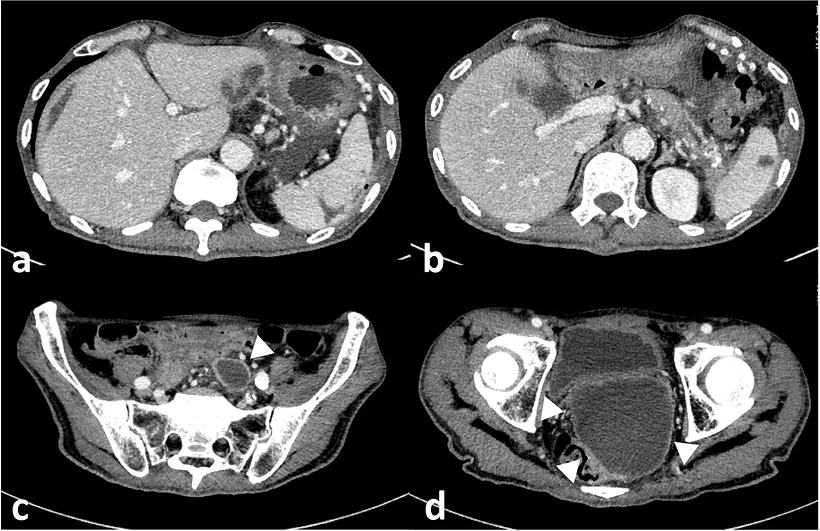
Contrast‐enhanced computed tomography findings at the onset of acute exacerbation of chronic pancreatitis. (a, b) Contrast‐enhanced computed tomography showing inflammatory changes around the pancreas with multiple pancreatic calcifications, consistent with chronic pancreatitis. Peripancreatic fluid collections are observed around the pancreatic body and tail. (c, d) Encapsulated pelvic fluid collections (arrowheads) are visible adjacent to the rectosigmoid colon and urinary bladder. These findings were considered compatible with pancreatitis‐related encapsulated peripancreatic and pelvic fluid collections. Because obvious necrotic debris was not clearly identified and the pelvic collection appeared relatively homogeneous, a pancreatic fluid leak‐associated or pseudocyst‐like collection was also considered.
